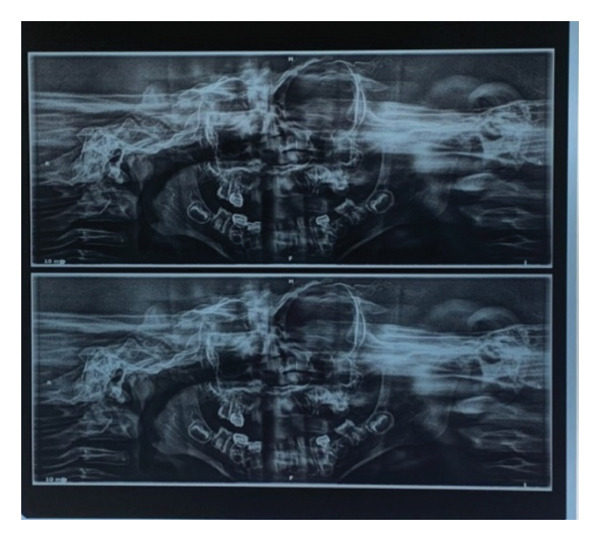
Intraoral photograph demonstrating severe dental manifestations. The image shows significant dental pathology, including extensive caries affecting all primary teeth, hypomineralization, and partially erupted permanent molars. Multiple periapical abscesses are present, consistent with the dental fragility observed in this connective tissue disorder.
